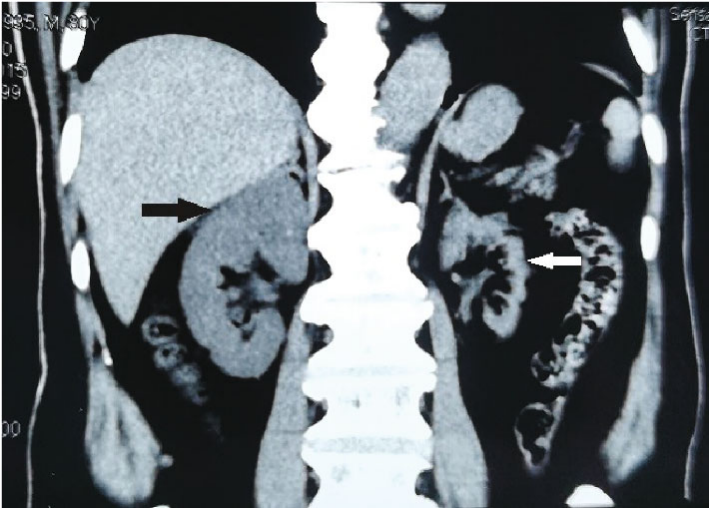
CT shows left renal atrophy, renal cortex thinning (white arrow), and right kidney compensatory hypertrophy (black arrow).
